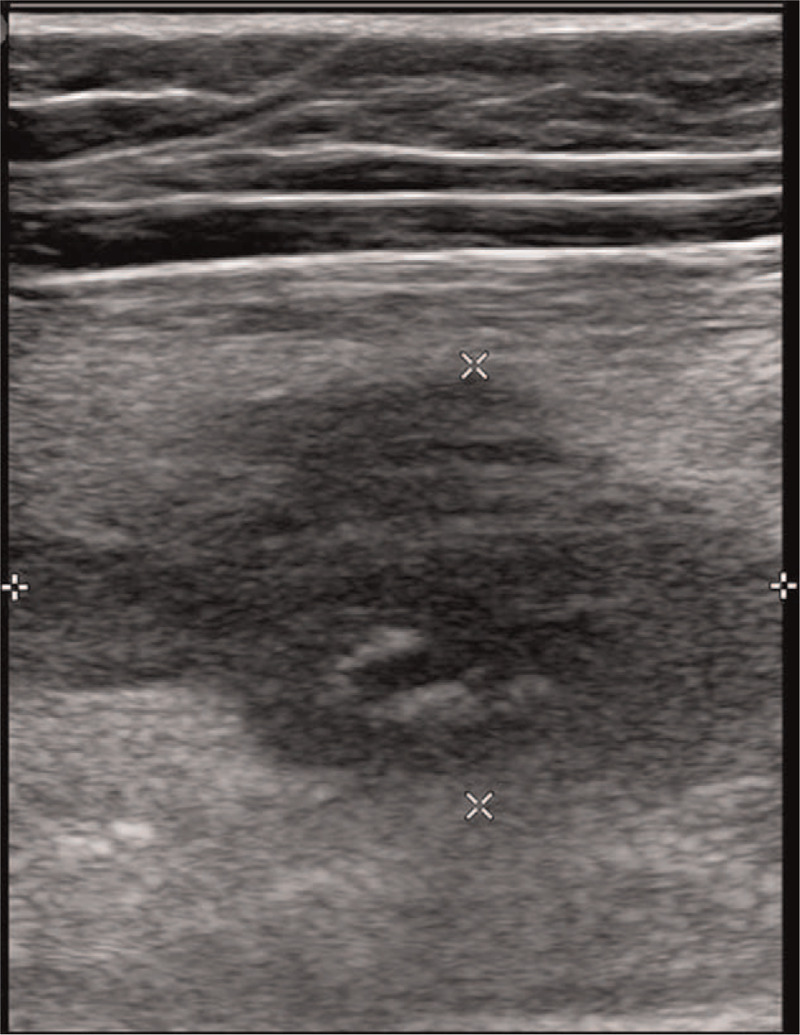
Musculoskeletal ultrasound of a 41-year-old female with right lower extremity pain and swelling. At the medial aspect of the thigh, there was an ill-defined hypoechoic mass-like lesion with peripheral calcification.
